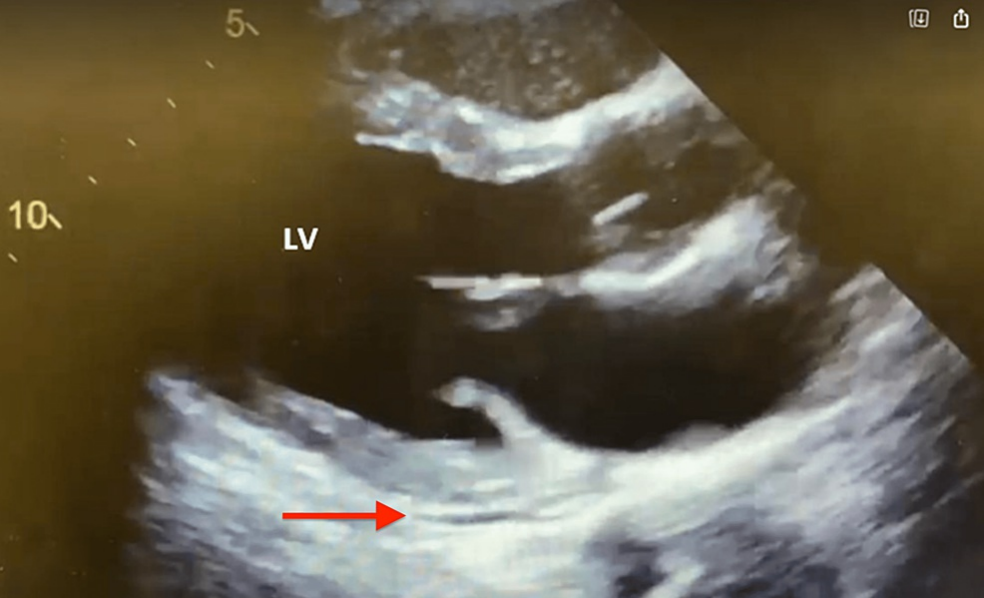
Initial echocardiogram on admission with LV EF 45-50%, mild hypokinesis, and trivial posterior pericardial effusion (red arrow) LV EF: left ventricular ejection fraction
